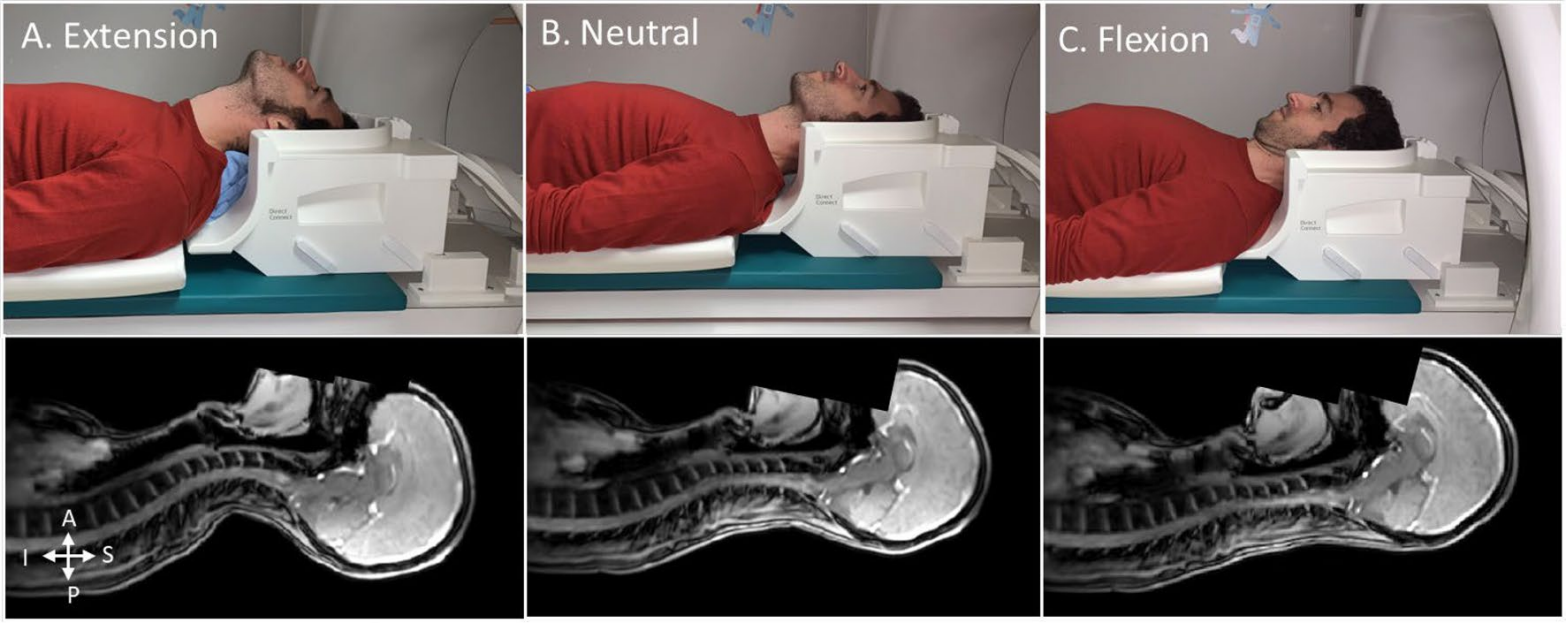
Example of the head positioning in the MRI’s head coil for neck extension (A), neutral (B) and flexion (C). The bottom row shows localizers. (One of the authors is in the scanner).
